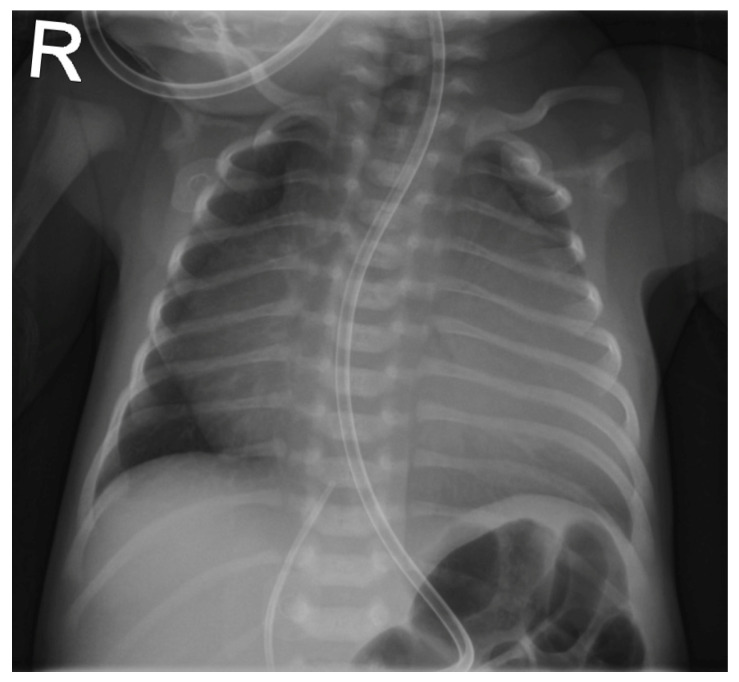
Chest radiograph showing severe cardiomegaly; immediately postpartum.
